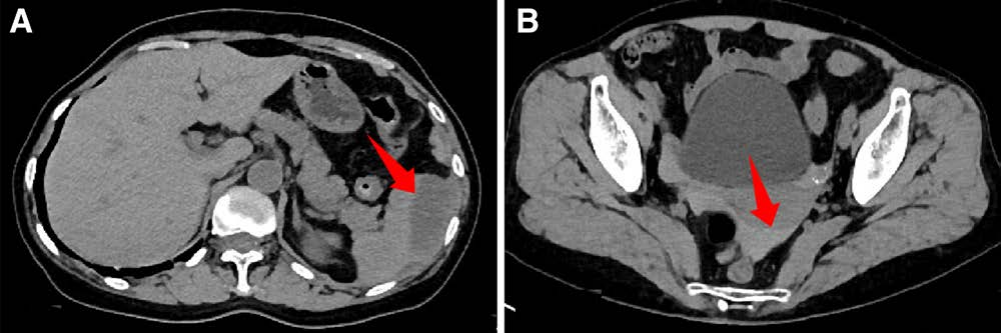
Postoperative day 9 CT images: (A) The arc-shaped low-density area under the splenic capsule has slightly increased in size (67 × 25 mm) with clear margins, representing a subcapsular hematoma. No active parenchymal bleeding is visible (blush sign). (B) The high-density area in the rectouterine pouch has slightly decreased (65 × 18 mm), indicating a pelvic hematoma. CT = computed tomography.
